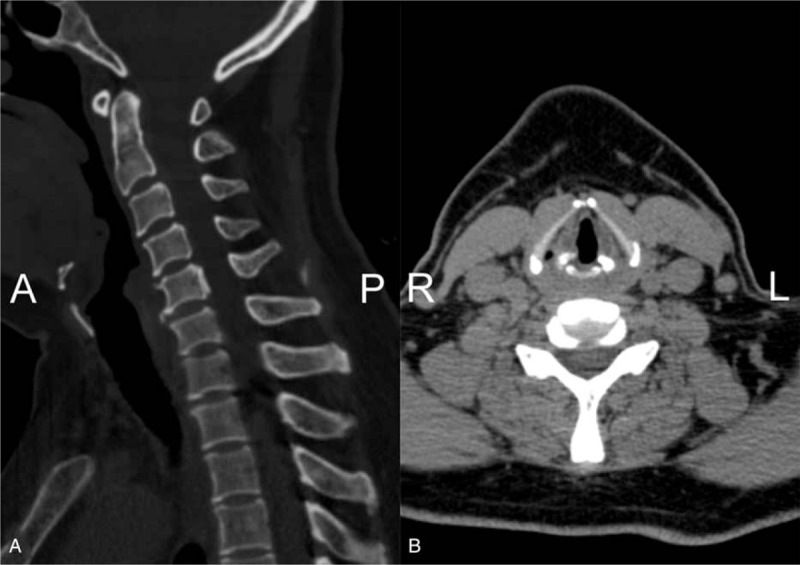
Sagittal (A) and axial (B) of cervical spine CT showed that the cervical curvature became straight.
